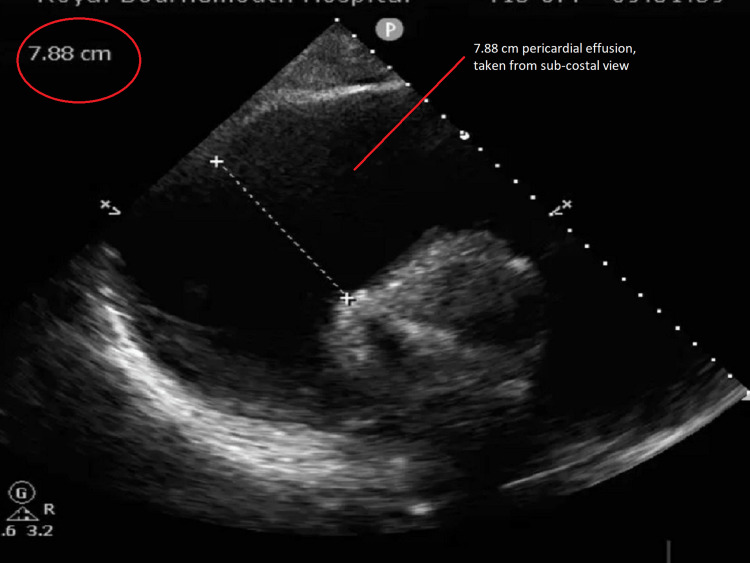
Transthoracic echo (subcostal view) Massive pericardial effusion measured up to 7.88 cm.
